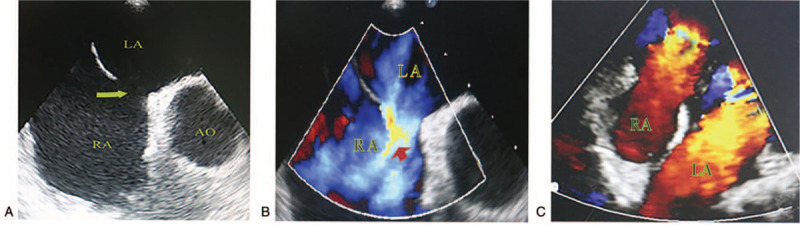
(A) Atrial septal defect (ASD) (arrow) was found on a transthoracic echocardiography. (B) Right-to-left shunts (RLS) were detected on color Doppler of transesophageal echocardiography (TEE) (arrow). (C) Postoperative TEE showed that the ASD was closed seamlessly, without blood flow. LA = left atrium, RA = right atrium, AO = aorta.
